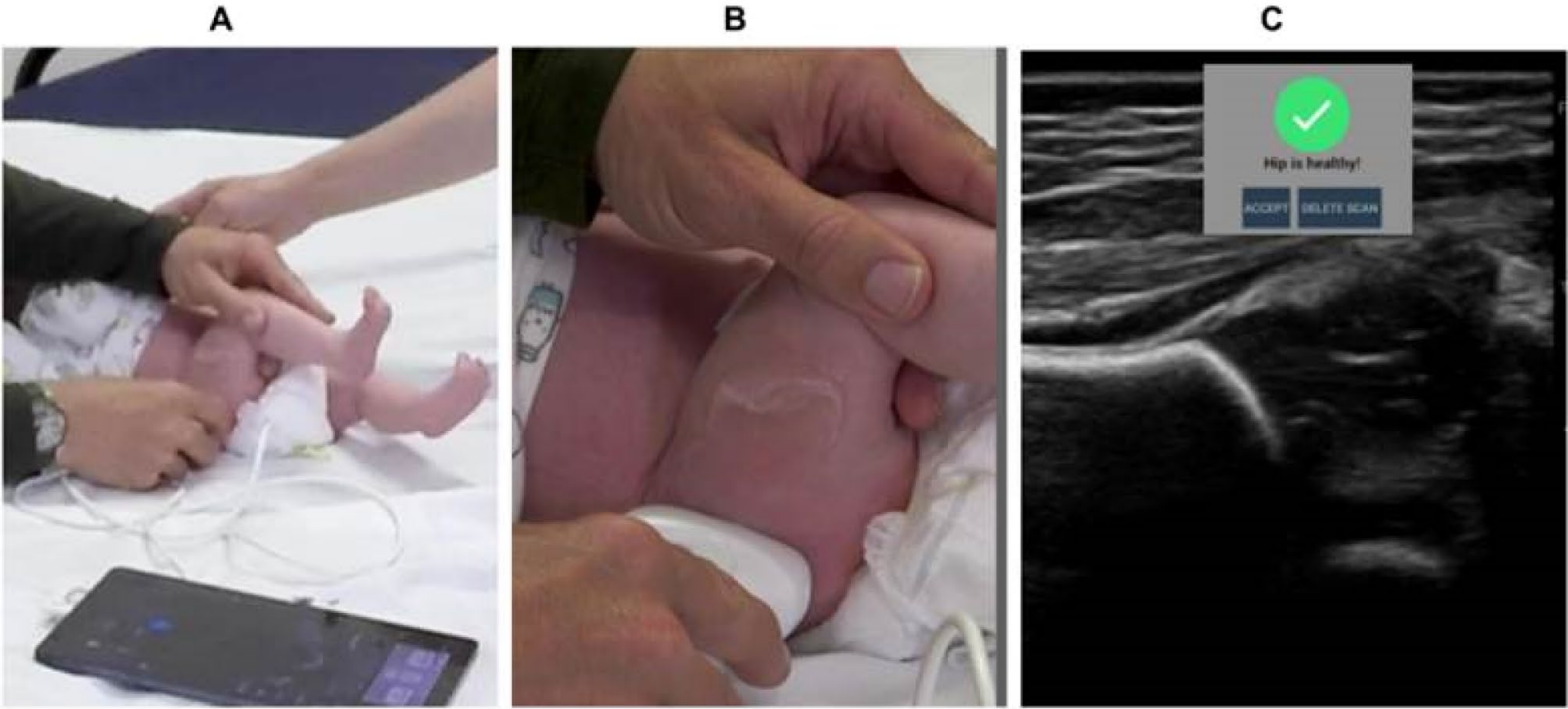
Scanning an infant’s right hip using handheld portable ultrasound probe and tablet (A and B), with Lumify and MEDO apps running (hip ultrasound image and app notification shown in C).
